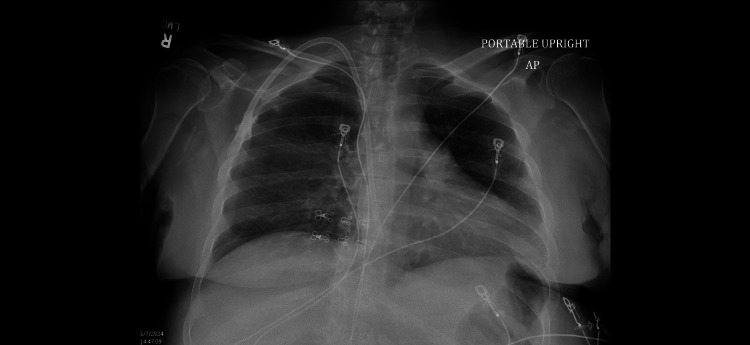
Chest X-ray on admission showing cardiomegaly with left pleural effusion without lung consolidation
